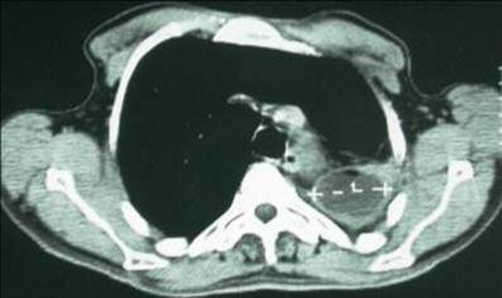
Computed tomography (CT) scan showing pleural pocket.
